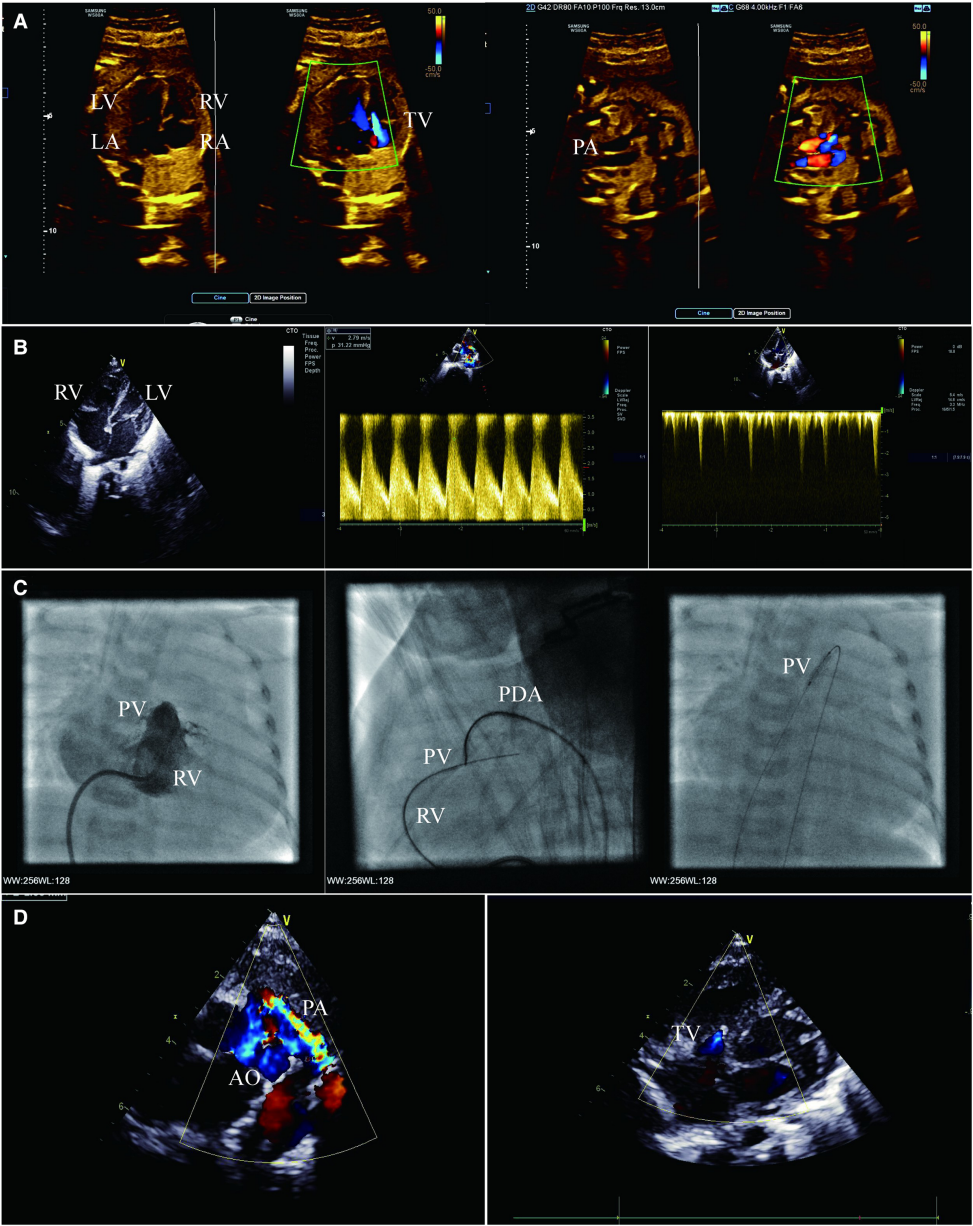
(A) Fetal echocardiogram apical four-chamber view (left) and right ventricular outflow tract view (right) showing severe pulmonary stenosis and massive tricuspid regurgitation. (B) Transthoracic echocardiogram apical four-chamber view (left and right) and short-axis view (middle) showing mild hypoplasia of RV, pulmonary atresia and patent ductus arteriosus, no serious abnormality of TV, and mild tricuspid regurgitation. (C) Right ventriculography (left) showing mild hypoplasia of RV and pulmonary valvular atresia. Lateral right ventriculography (middle) showing guide wire creating an arteriovenous wire loop. Anterior chest fluoroscopy (right) showing percutaneous balloon pulmonary valvuloplasty. (D) Transthoracic echocardiogram short-axis view (left) and apical four-chamber view (right) showing ductus arteriosus supply pulmonary blood flow, no antegrade blood flow across the pulmonary valve, and mild tricuspid regurgitation. LA, left atrium; LV, left ventricle; PV, pulmonary valve.
